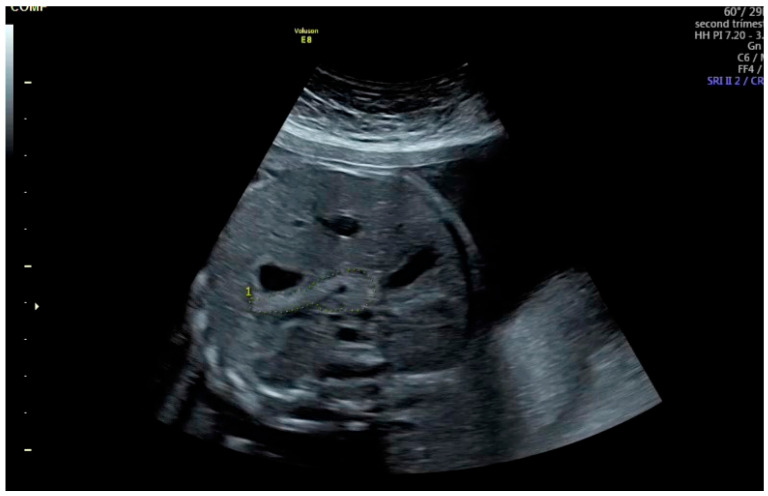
Gray scale ultrasound showing fetal pancreas circumference with dotted lines. The pancreas is seen as a slightly echogenic structure behind the stomach, anterior to the left kidney, aorta, vena cava superior and vertebral spine, and extending to the gallbladder on the right.
